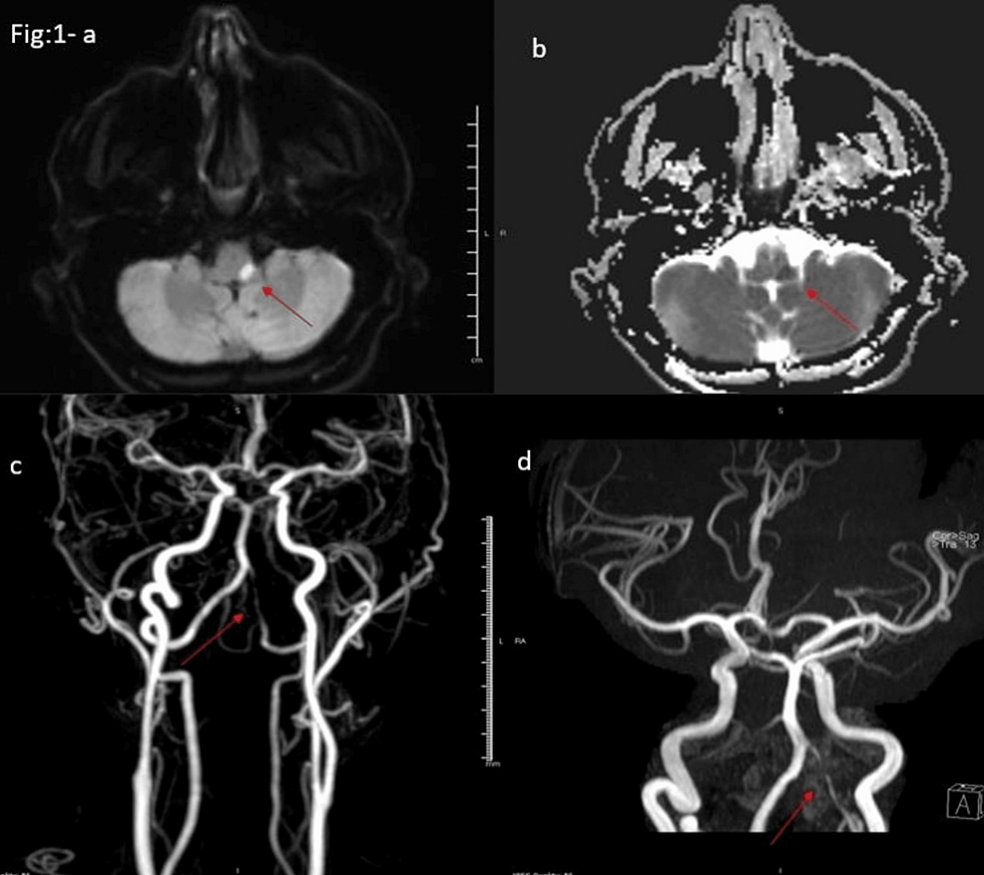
Head MRI and MRA of the head and neck vessels depicting a left lateral medullary stroke and left vertebral artery atherosclerotic disease. Head MRI images (a and b) reveal an acute left lateral medullary infarct. MRA of the head and neck (c and d) demonstrate severe atherosclerotic disease in the left vertebral artery, as indicated by the red arrow.
